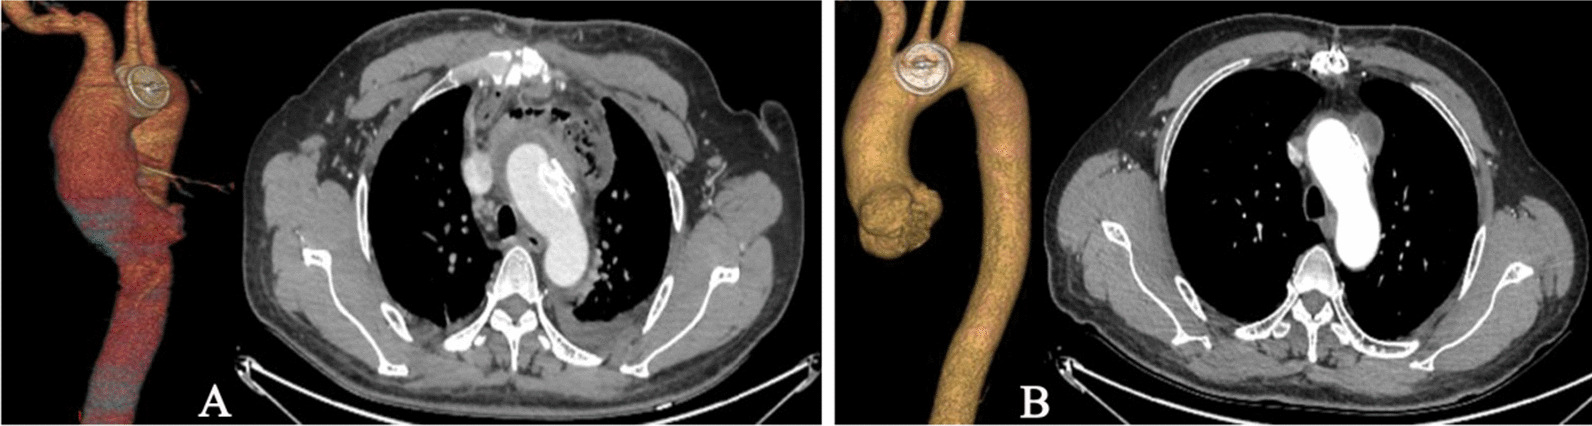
Postoperative imaging. A 1 month after discharge. B 7 months after discharge. The CTA images show that the occluder was placed well and had a normal morphology, without aortic dissection
